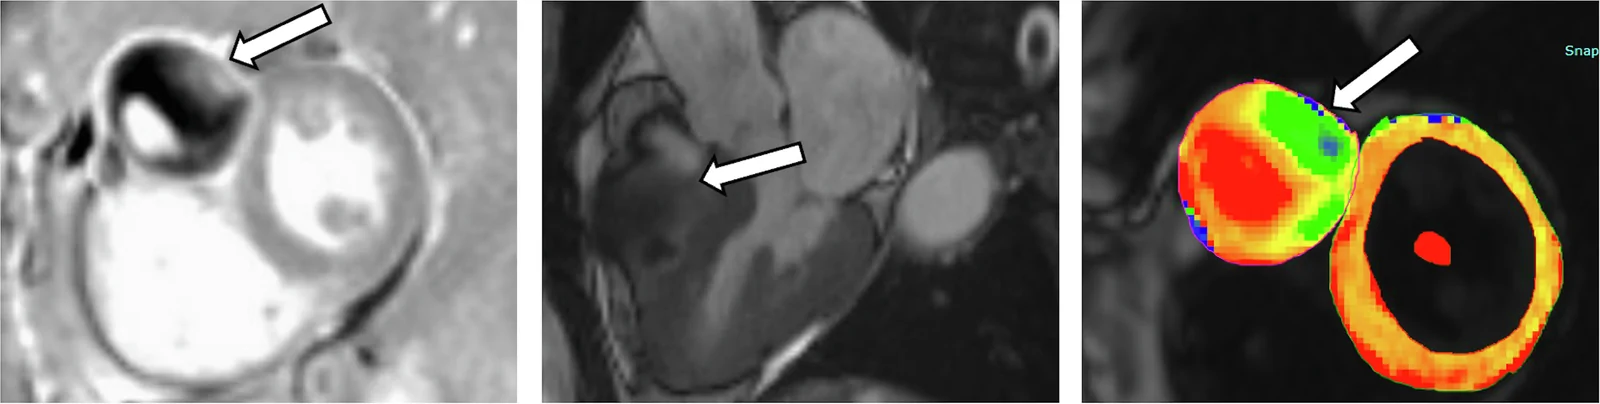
Magnetic Resonance Imaging of Coronary Aneurysm Cardiac magnetic resonance with post-gadolinium contrast inversion recovery and T1 parametric mapping demonstrating a mass in the anterior interventricular groove, containing calcium, thrombus, and blood pool (arrows).
